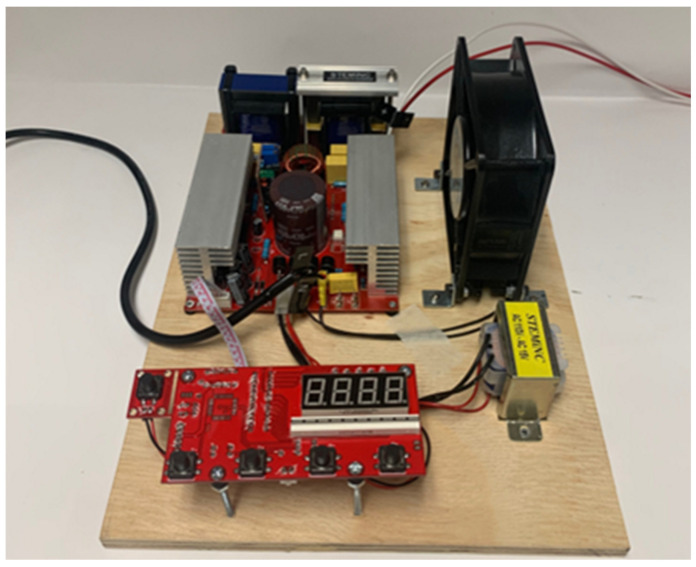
Ultrasonic power generator.
